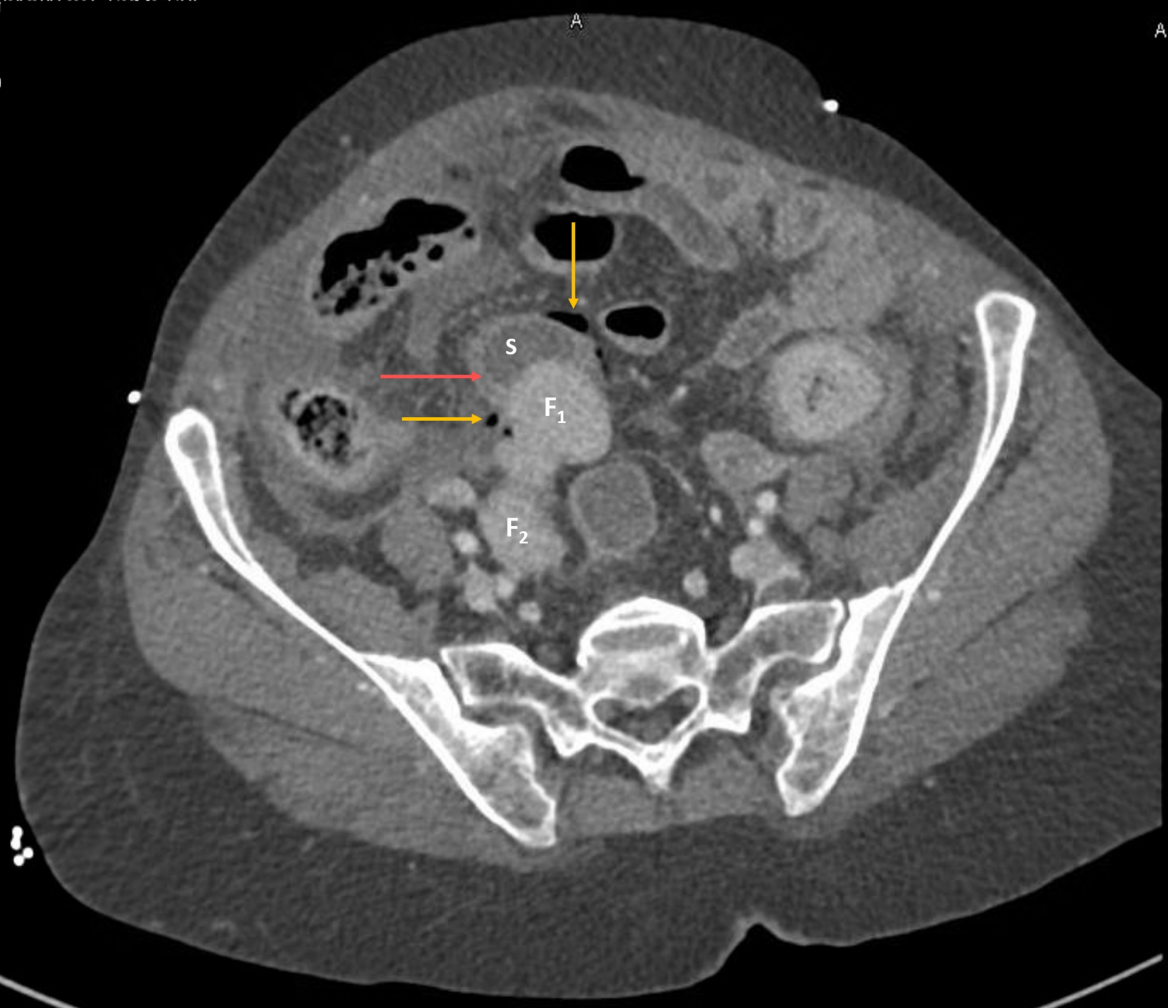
Contrast-enhanced CT scan of the abdomen, axial view F_1_ and F_2_ are faecalomas. S shows sigmoid with the bowel wall shown with red arrow. Fecaloma F_1_ is seen disrupting the sigmoid lumen continuity causing extraluminal free air (marked with yellow arrows) adjacent to the sigmoid. Fecaloma F_2_ is extraluminal and lying in the peritoneal cavity
